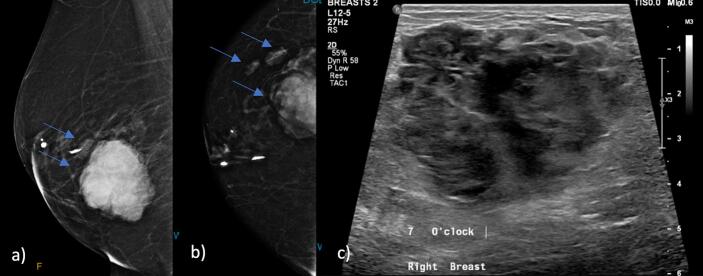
Right breast Mammogram a) Medial Lateral Oblique (MLO) projection and b) cranial caudal (CC) projection showing multiple breast lesions (blue arrow). Right breast ultrasound c) heterogonous mass. (For interpretation of the references to colour in this figure legend, the reader is referred to the web version of this article.)
